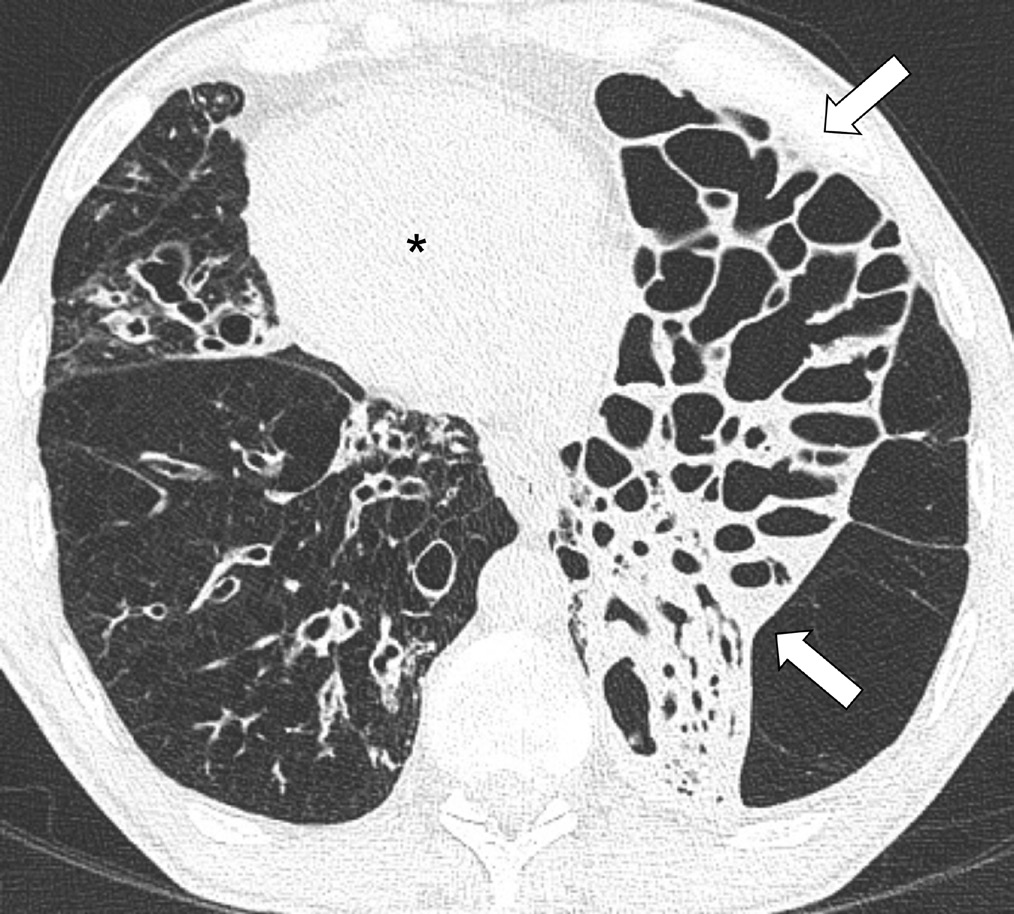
Axial CT image of a patient diagnosed with Kartagener syndrome shows dextrocardia (asterisk) and bilateral bronchiectases involving the middle lobe and both lower lobes. Marked volume loss is evident in the left lower lobe (arrows).
